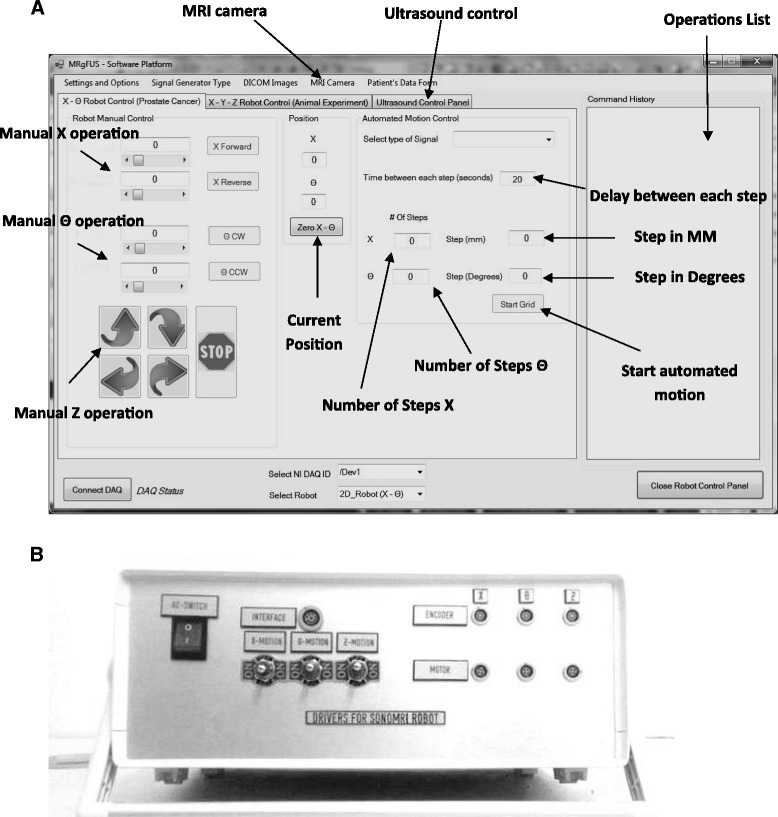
Main window of the software and electronic system. (A) Software used to control the positioning device and the therapeutic system which is guided by MRI, and (B) electronic system.
